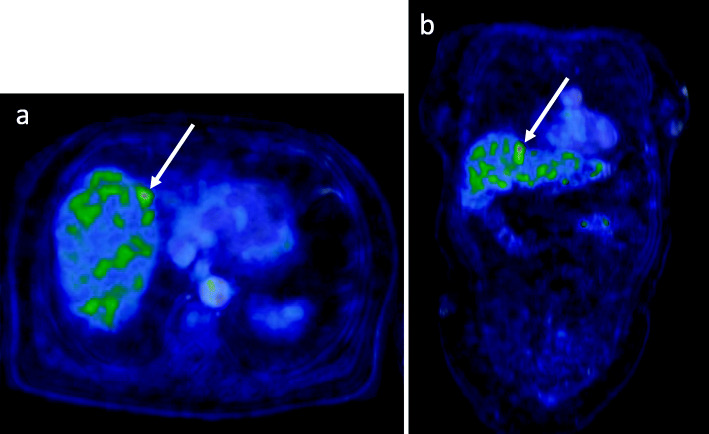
A 60-year old woman with a locally advanced disease and a small FDG avid liver metastasis in segment IV (white arrows). Whole-body PET/MRI images (with T1-weighted 3D water Dixon MRI) in axial (a) and coronal (b) plane
